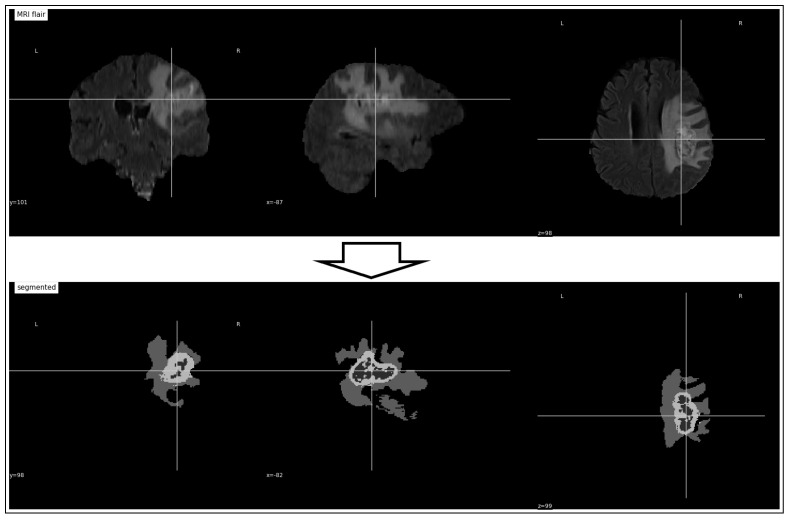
Example of the segmented tumor from the MRI.
